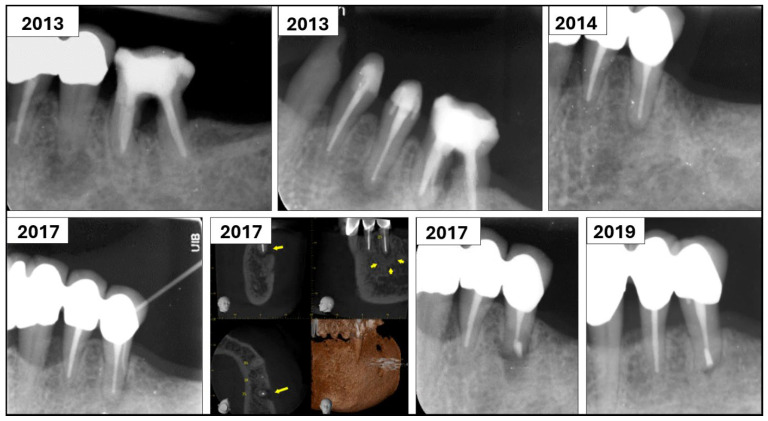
Case 3 belonged to a 67-year-old patient who was diagnosed with necrotic pulp and chronic apical periodontitis on tooth 35. Following cleaning, shaping, and intracanal dressing for two weeks, the root canal was filled with gutta-percha and AH-plus sealer. At one-year recall, there were no symptoms from the tooth, and apical radiolucency had healed. However, in 2017, the tooth was diagnosed with periapical abscess with sinus tract. A cone beam CT image showed a periapical lesion (marked with yellow arrows). Endodontic surgery was performed on the tooth at the postgraduate clinic. Recall from 2019 showed resolution of the periapical lesion and the tooth was symptom-free.
